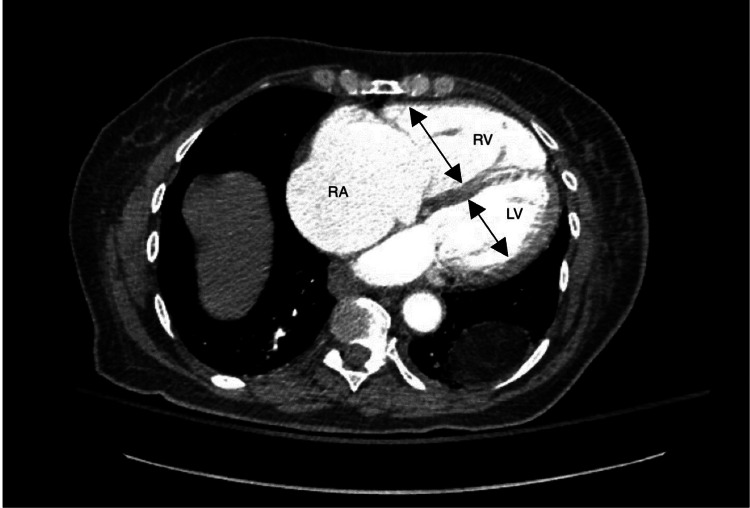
CT angiogram abdomen/pelvis: cardiac cut Marked dilation of the right ventricle (RV) and right atrium (RA), indicative of severe RV dysfunction. Compare with the relatively smaller left ventricle (LV), highlighting the disproportionate right-sided chamber enlargement seen in RV failure.
